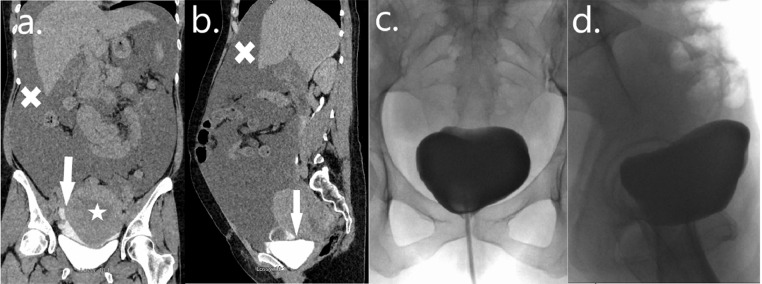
Case 2—Iatrogenic intraperitoneal bladder perforation: Coronal (A) and sagittal (B) images from a CT urogram demonstrating contrast extravasation (arrows) with a large volume of intraperitoneal-free fluid (crosses) and a thickened postpartum uterus (star). Anterior-posterior (C) and lateral (D) images of a retrograde stress cystogram confirming no residual urine leak following conservative management with an indwelling urethral catheter.
